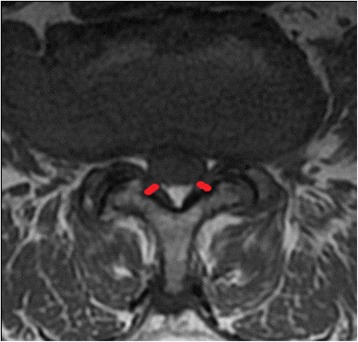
Measurement of ligamentum flavum (LF) thickness using T2-weighted axial MRI scan (marked in red lines) - at the cut with the thickest LF measured at the midpoint of the facet joint level
